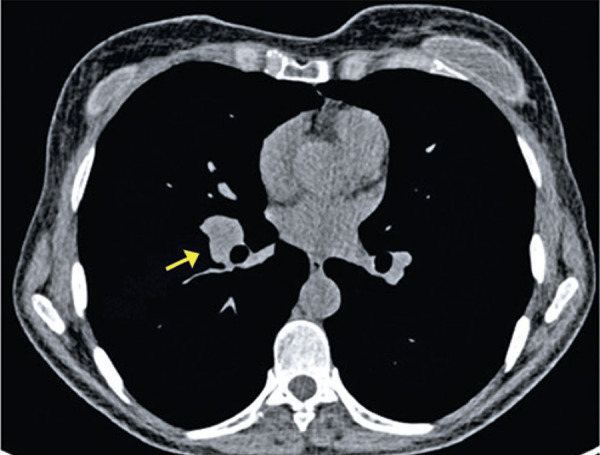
A 52-year-old patient with COVID-19, presenting dyspnea, fever, and myalgia for three days. Computed tomography shows hilar lymphadenopathy on the right (arrow)
